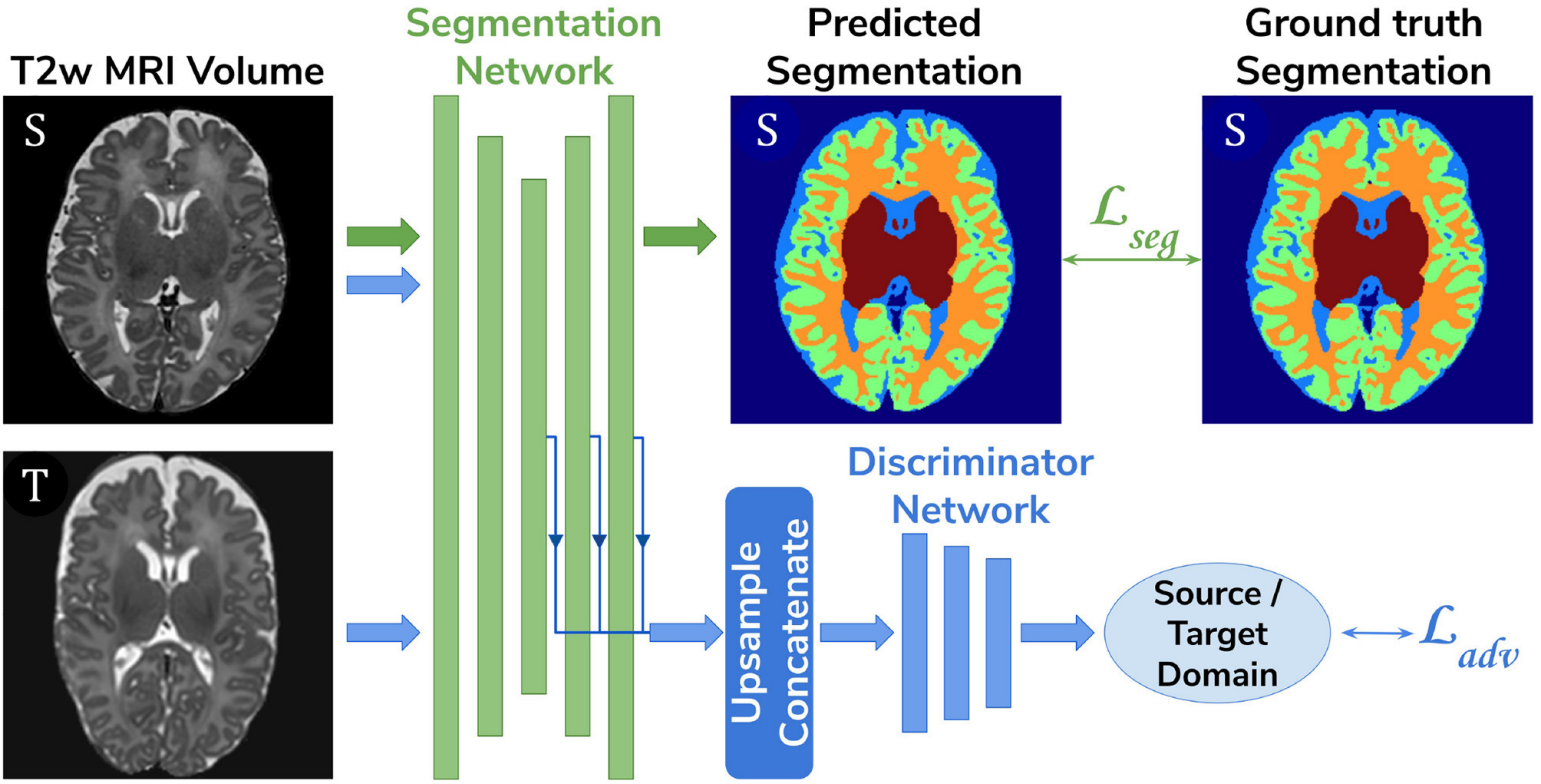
The latent space domain adaptation setup consists of a 3D U-Net trained to segment the source (dHCP) *T*2w MRI volumes, coupled with a discriminator network which forces the segmentation network to learn domain-invariant features. Both source (dHCP) and target (ePrime) images are fed to the segmentation network, but only source (dHCP) Draw-EM output labels are used to compute the segmentation loss. Source domain images are marked with S, while target domain images are marked with T, respectively.
